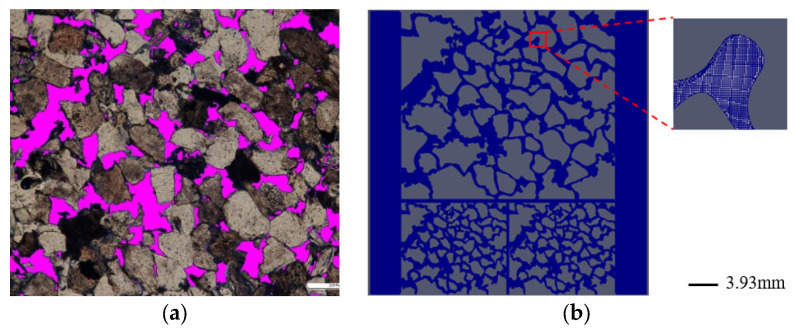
Model of a complex dual heterogeneous pore: (a) CT scan image; (b) mesh profile of a two-layer heterogeneous model.
